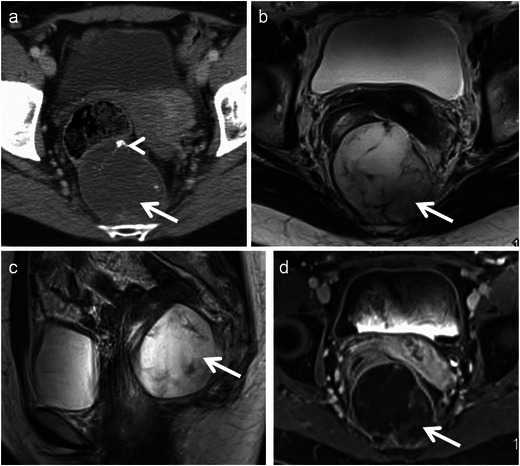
Sub peritoneal pelvic adenomucinosis presenting as slow growing pelvic mass 6 years after surgery for appendiceal mucocele. a Axial contrast CT reveals retrorectal multiloculated cystic lesion (*arrow*) with calcification (*arrowhead*). b Axial T2-weighted MRI and c sagittal T2-weighted MRI reveal a cystic lesion (*arrow*) with multiple septations. d Axial contrast T1-weighted MRI reveals peripheral and septal enhancement of the lesion (*arrow*)
